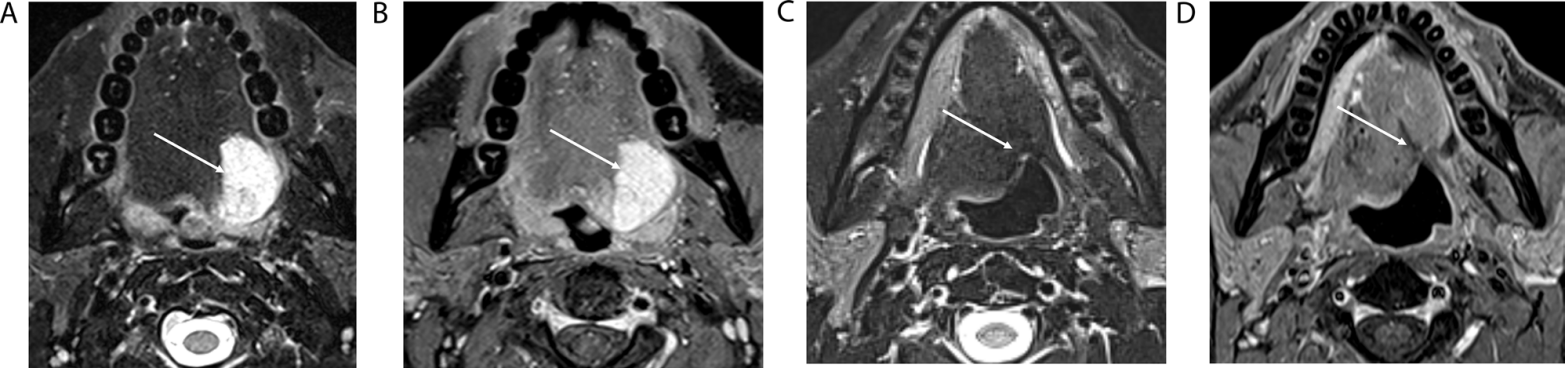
Axial T2-weighted fat-suppressed (A) and axial T1-weighted fat-suppressed post contrast (B) MR images of an adenoid cystic carcinoma (arrows) of the left base of tongue at initial staging. Axial T2-weighted fat-suppressed (C) and axial T1-weighted fat-suppressed post contrast (D) MR images of the same patient following definitive treatment with resection and adjuvant radiation therapy with features of NI-RADS MRI primary category 1 including non-mass-like distortion of soft tissues with low T1 and low T2 signal intensity suggesting scar/fibrosis (arrows)
